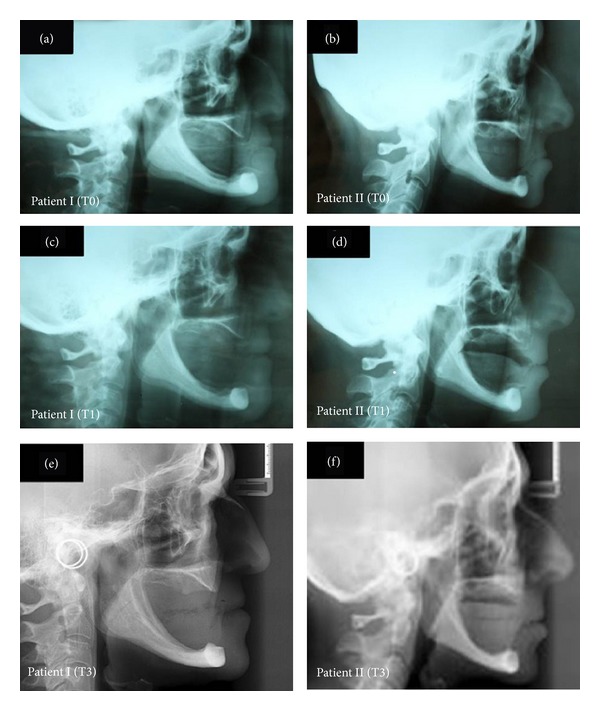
Lateral cephalometric radiograph of patients. (a) Patient I at baseline or T0. (b) Patient II at baseline or T0. (c) Patient I at trial insertion or T1. (d) Patient II at trial insertion or T1. (e) Patient I after four years of rehabilitation or T3. (f) Patient II after four years of rehabilitation or T3.
